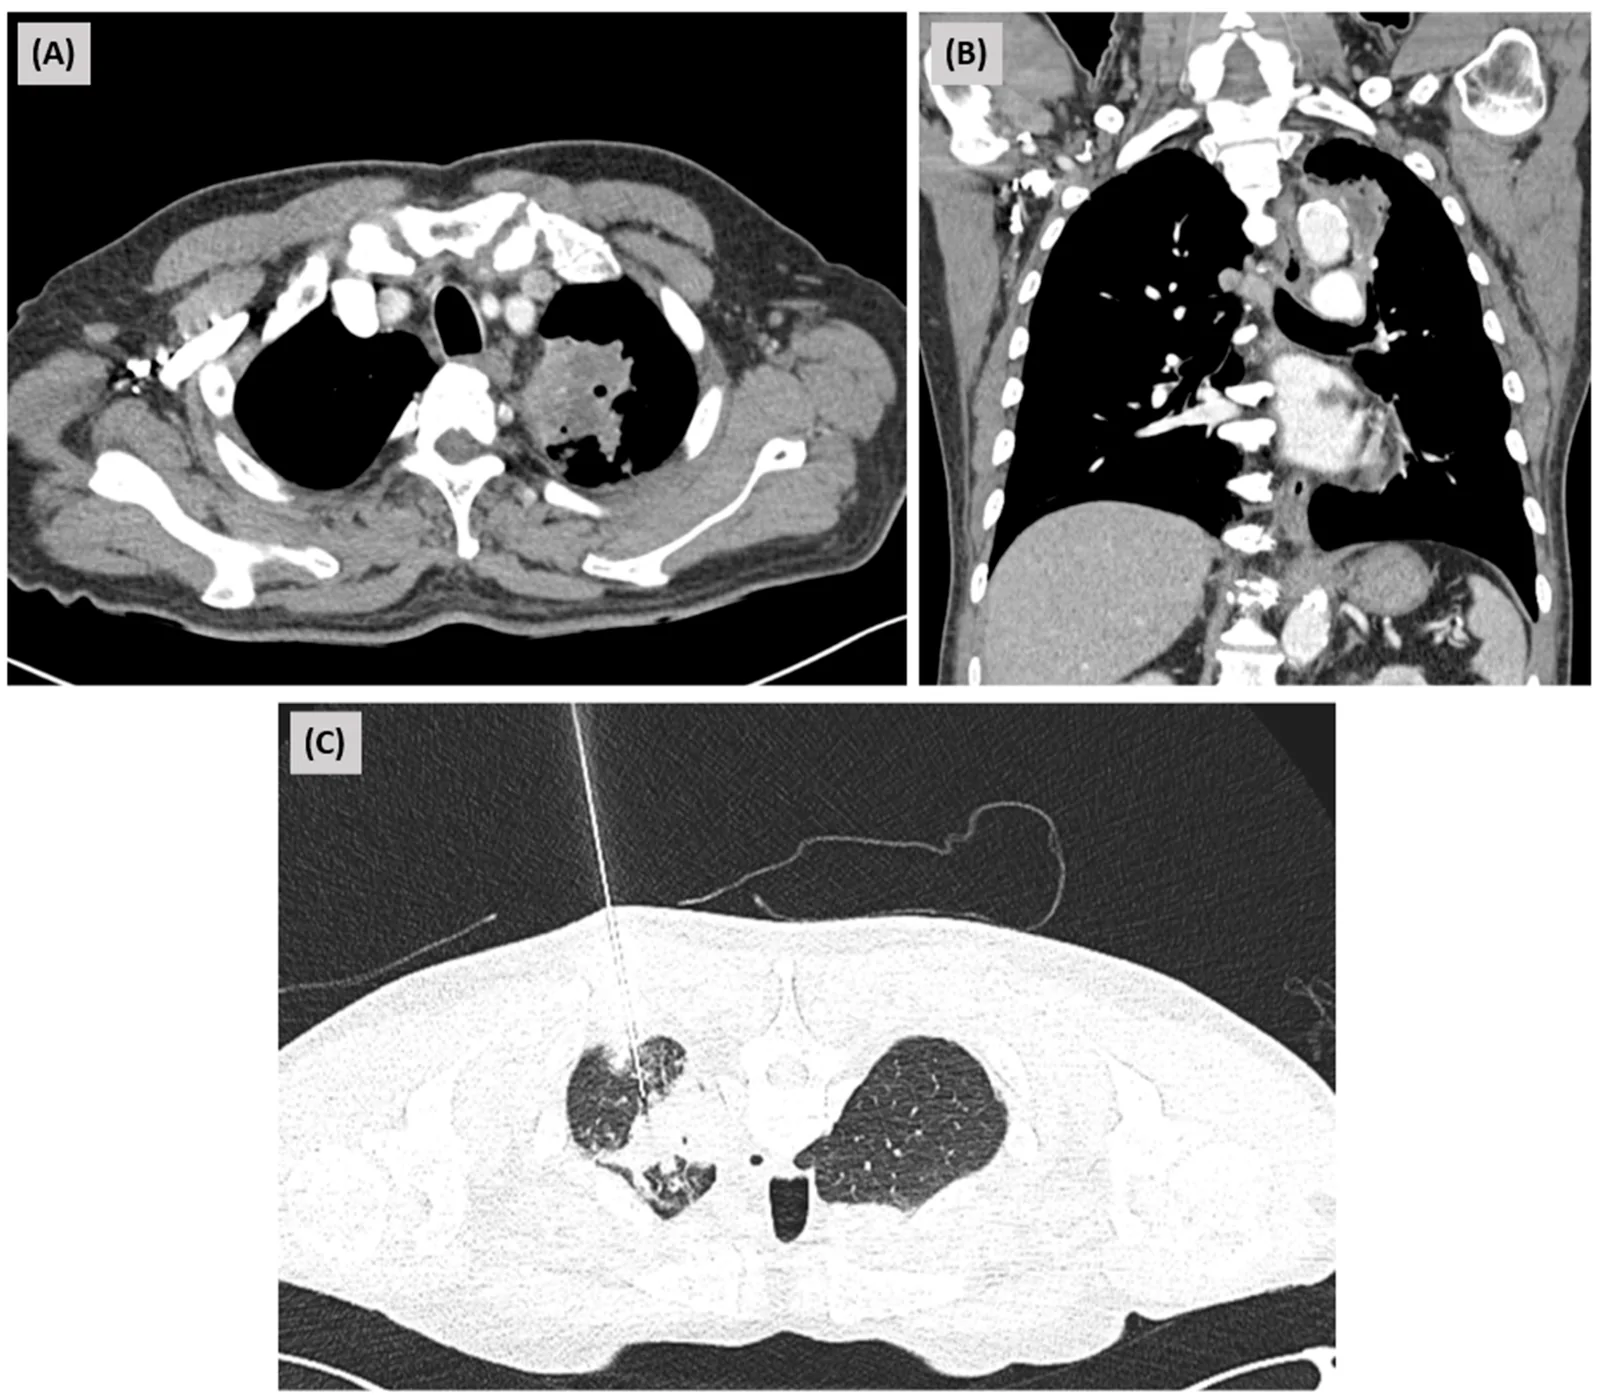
Initial imaging findings and biopsy procedure at an outside hospital. (A) Axial contrast-enhanced chest CT image demonstrating a mass-like lesion in the left upper lobe. (B) Coronal reconstructed CT image showing the extent of the lesion in the left upper lobe. (C) CT-guided percutaneous core needle biopsy performed for tissue diagnosis of the lesion.
